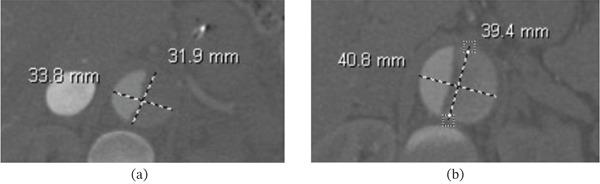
Surveillance CT scan at 1‐year follow‐up demonstrated aneurysmal degeneration of the aortic dissection from (a) 34 × 32 to (b) 41 × 39 mm.
